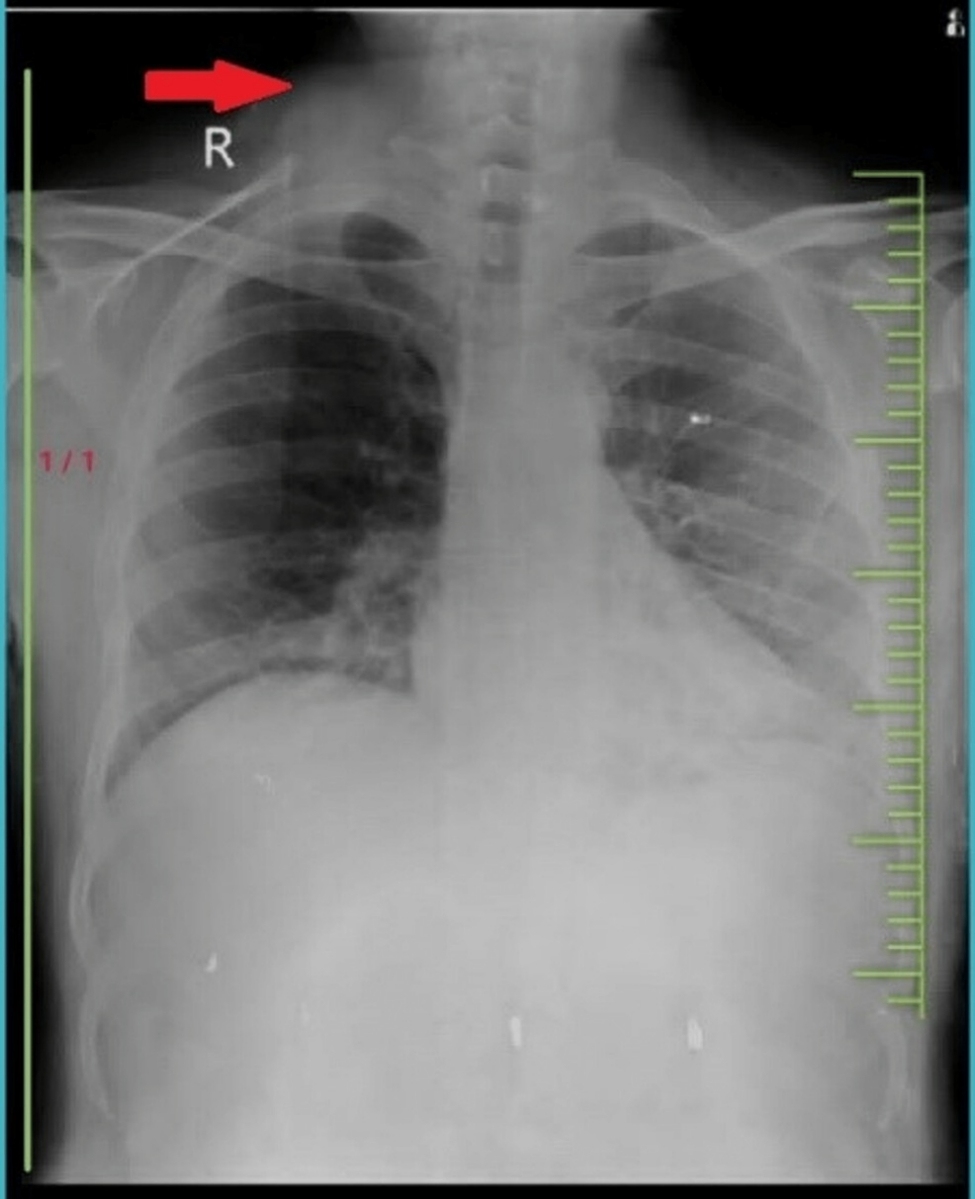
X-ray showing a mass in the right supraclavicular region with no bony involvement.
